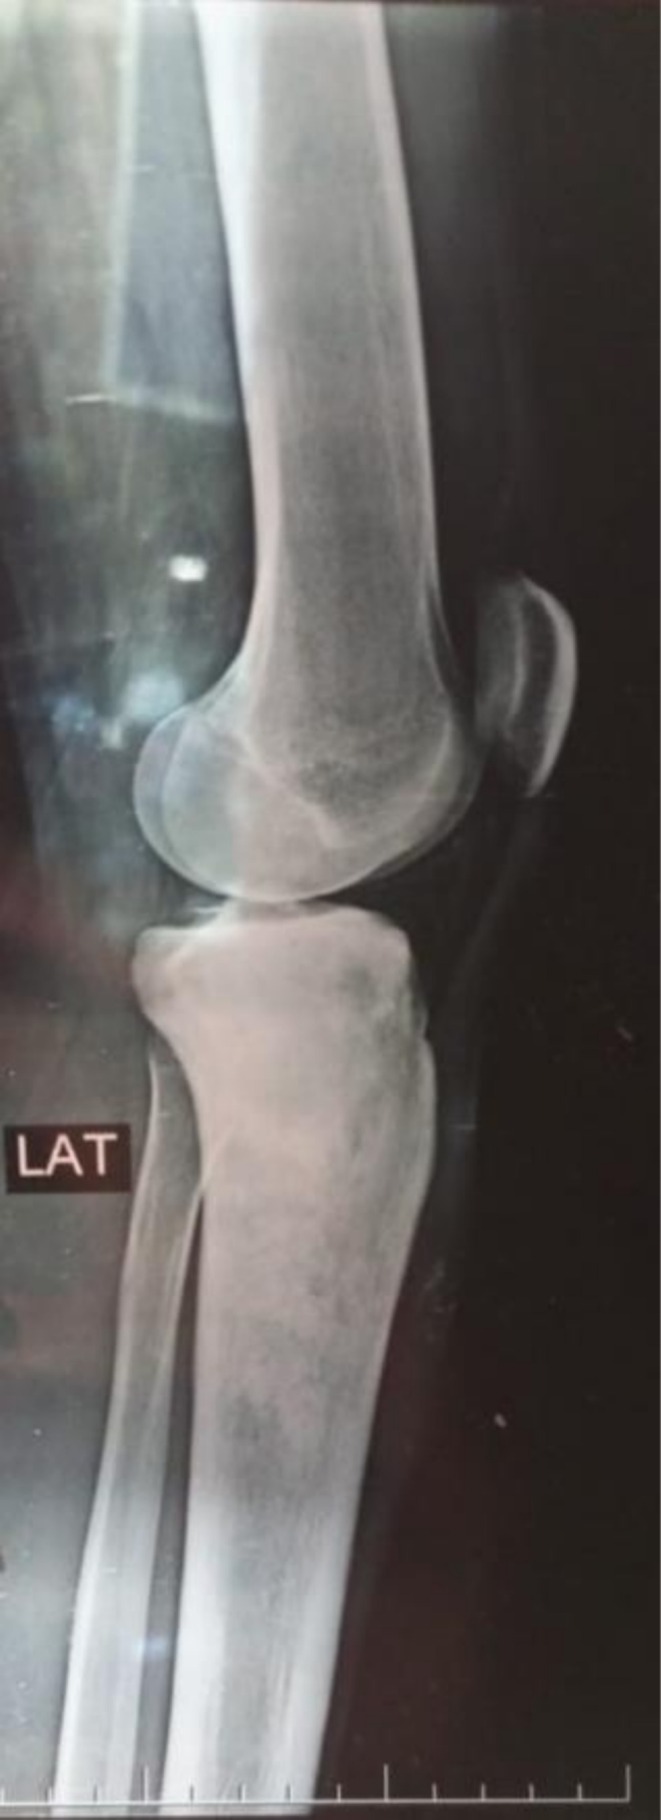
Lateral plain radiograph (X‐ray) of the right knee at presentation. This lateral view reveals a poorly defined, mixed lytic and sclerotic lesion in the proximal tibial metaphysis with typical “sunburst” periosteal reaction, findings highly suggestive of a primary malignant bone tumor. A significant associated soft tissue mass is visible, indicating extensive extra‐osseous tumor extension, a key finding for surgical planning.
